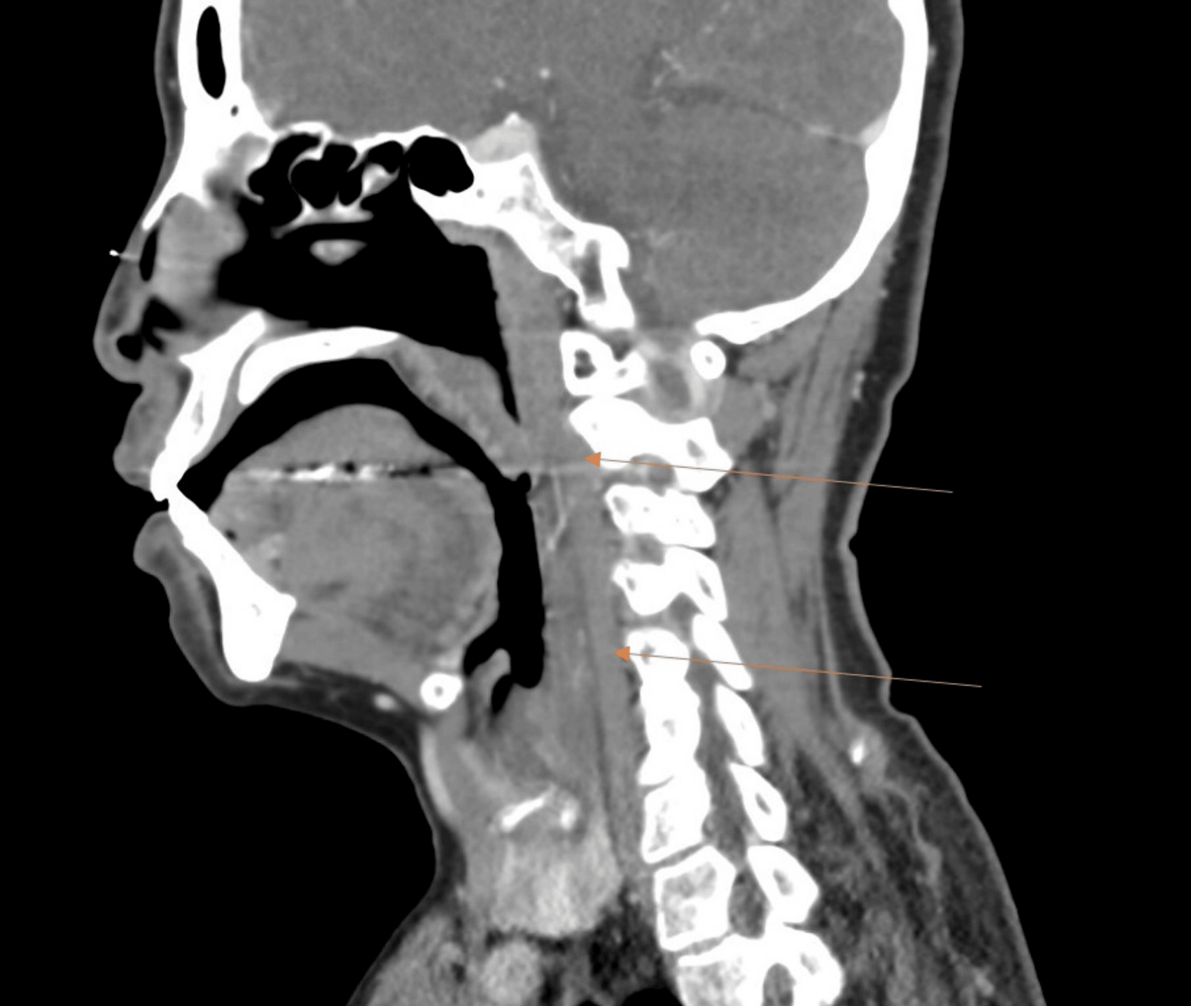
CT neck soft tissue with contrast: retropharyngeal edema from C2–C5. Superior arrow demonstrating start of edema. Inferior arrow demonstrating end of edema.
